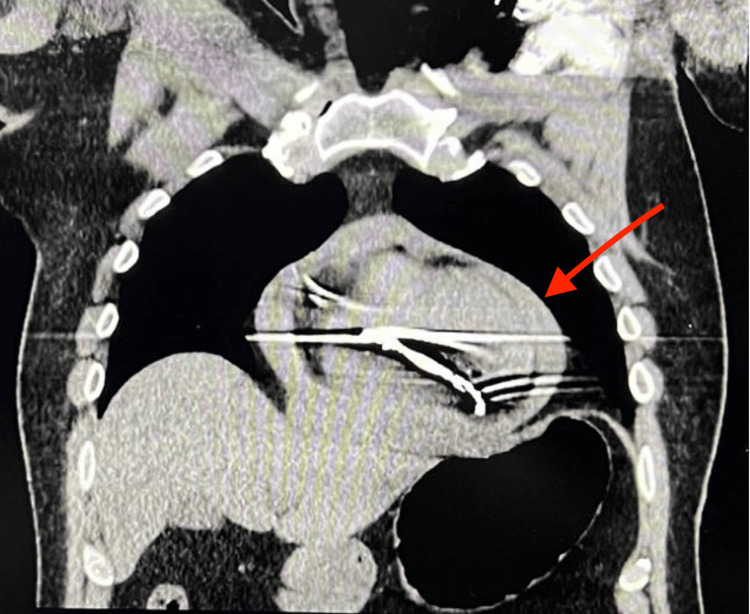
Computed tomography (CT) imaging obtained following the patient being admitted to the emergency department, confirming large pericardial effusion
